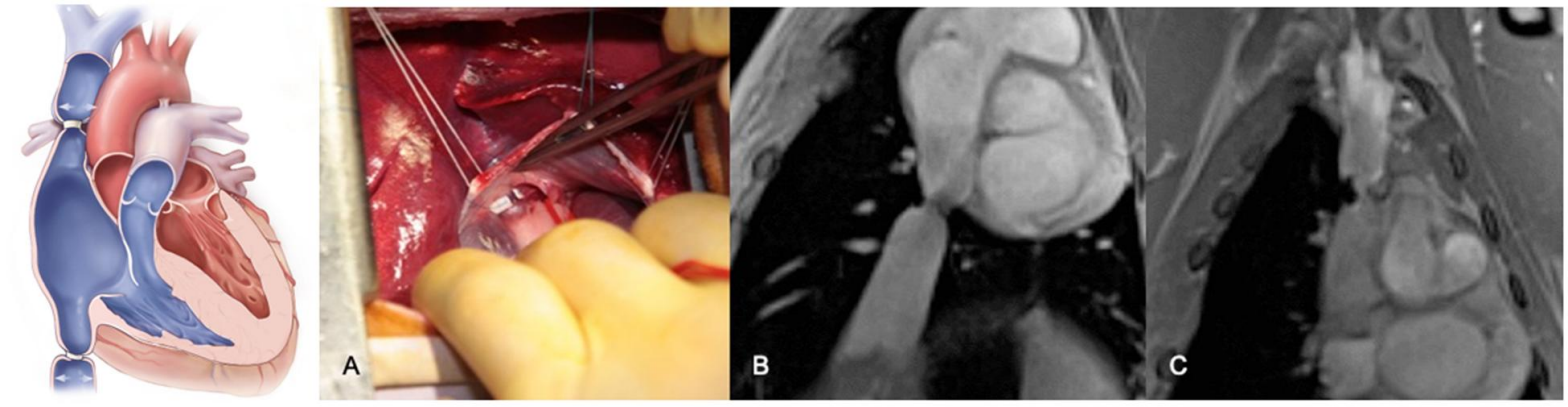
Banding of caval veins: (A) surgical view of the band (PTFE strip) of the SVC; (B) MRI showing the band of the IVC before debanding and (C) same for SVC.
